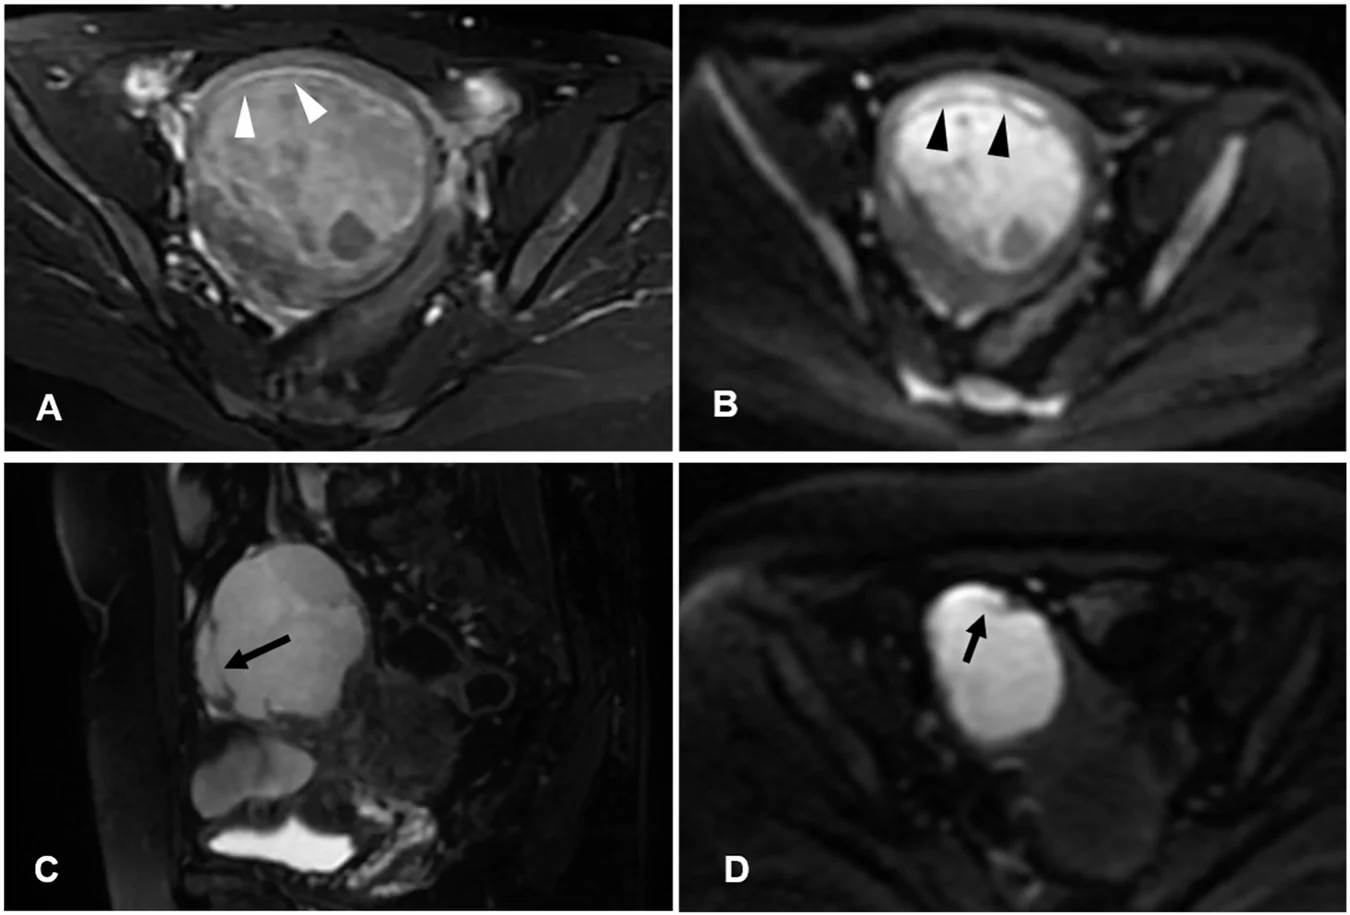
Magnetic resonance images of LG-ESS in a 52-year-old woman (A, B) and a 58-year-old woman (C, D). Axial T2W (A) and DW (B) images showing a well-defined, hyperintense tumor. Note the intratumoral T2WI hypointense band near the tumor margin (arrowheads). Sagittal T2W (C) and axial DW (D) images demonstrating an intratumoral T2WI hypointense band near the tumor margin (arrows)
